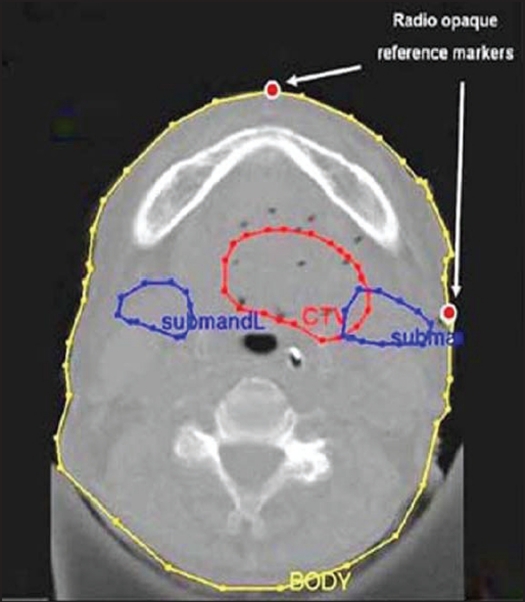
CT image containing the contours and radio-opaque reference markers
